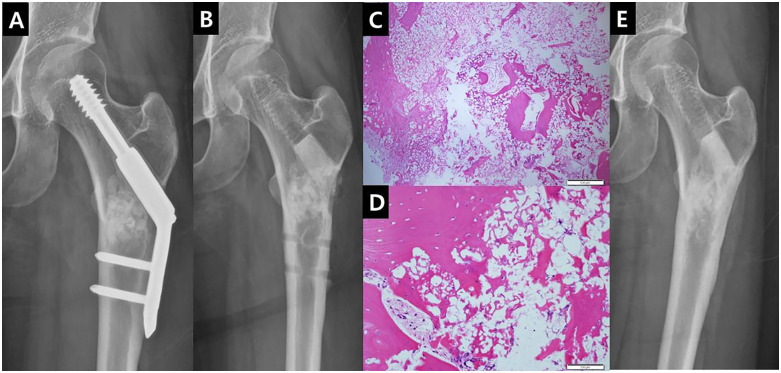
Benign bone tumor fixation case with pathologic reviews. A: This one-year postoperative photograph of a patient, who underwent curettage, pure β-TCP (Neobone^®^) grafting, and 2-hole compressive hip screw fixation for left femur subtrochanteric nonossifying fibroma, demonstrates the healing of the benign bone tumor. B: Implant removal was performed simultaneously with scar revision, and the area around the Lag screw hole was curettaged and filled with a 13 mm cylindrical pure β-TCP (Neobone^®^) bone substitute graft. A biopsy was taken from the previously curettaged surgical site. C: This low-magnification photograph shows dense fibrous collagen tissue on the outer left and the structure of pure β-TCP (Neobone^®^) just inside. Progressing right, the transition from woven bone in the middle to mature bone on the lower right is visible. D: In this high-magnification photograph, the sequence from the pure β-TCP (Neobone^®^) to the bone -forming part from the lower right to the upper left to the mature bone is depicted. The lamellar structure and the small cells, likely osteocytes, are clearly visible. E: An X-ray taken 1 year after the final surgery shows that the grafted pure β-TCP (Neobone^®^) bone substitute has contributed to host bone formation and cortical healing.
